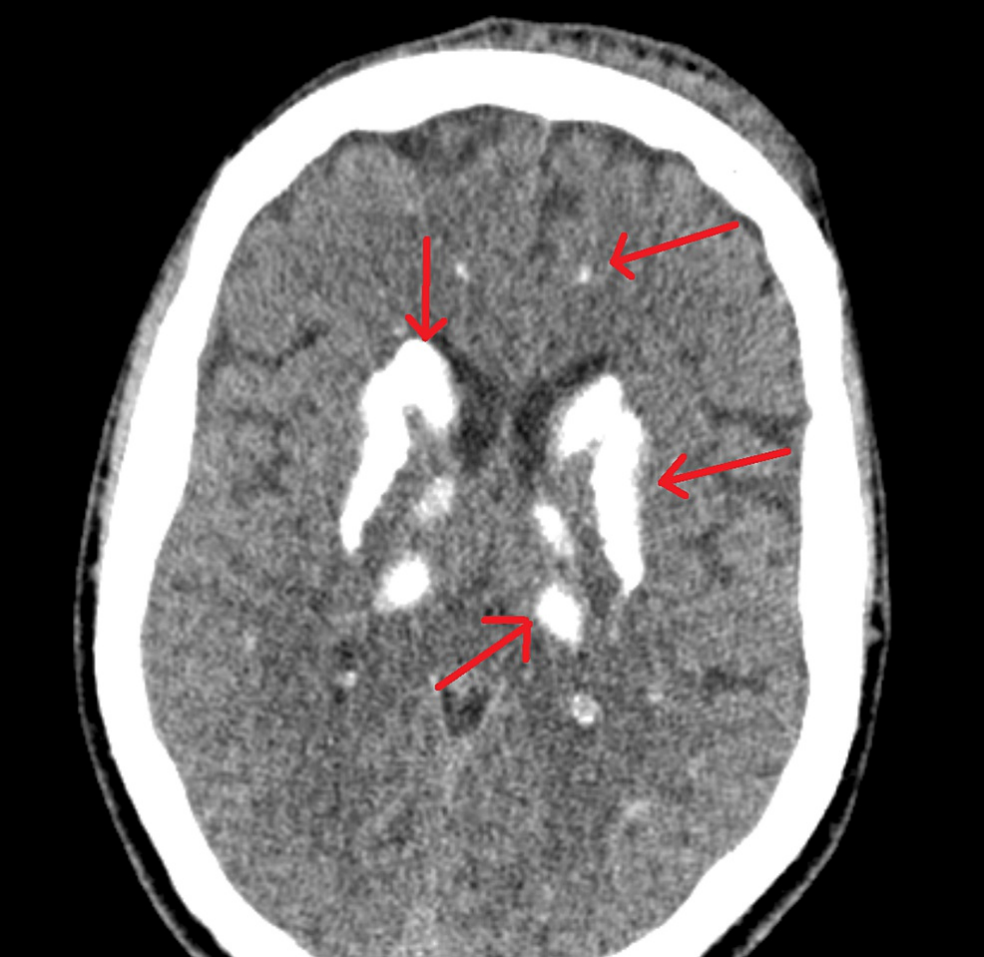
CT scan (axial plane). The arrows show calcifications on the putamen, globus pallidus, the tentorium itself and small calcifications in the frontal lobes and the thalamus.
